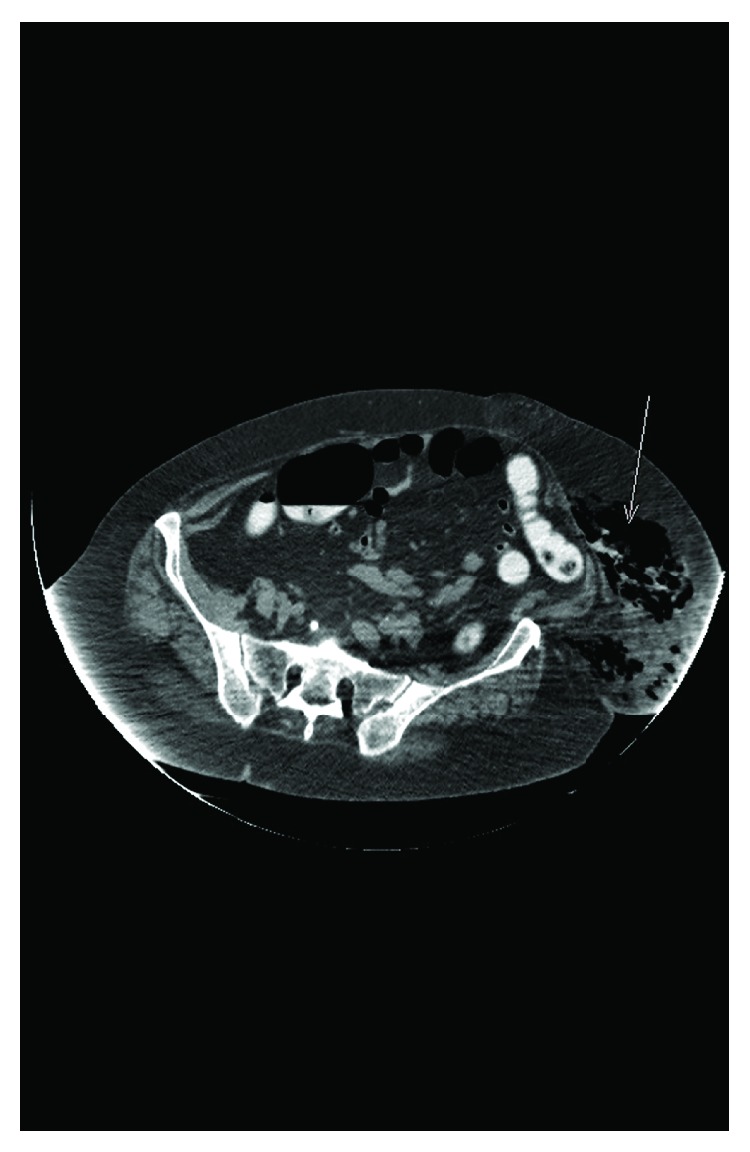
CT findings of the second case. The arrow represents once again the extraperitoneal perforation.
